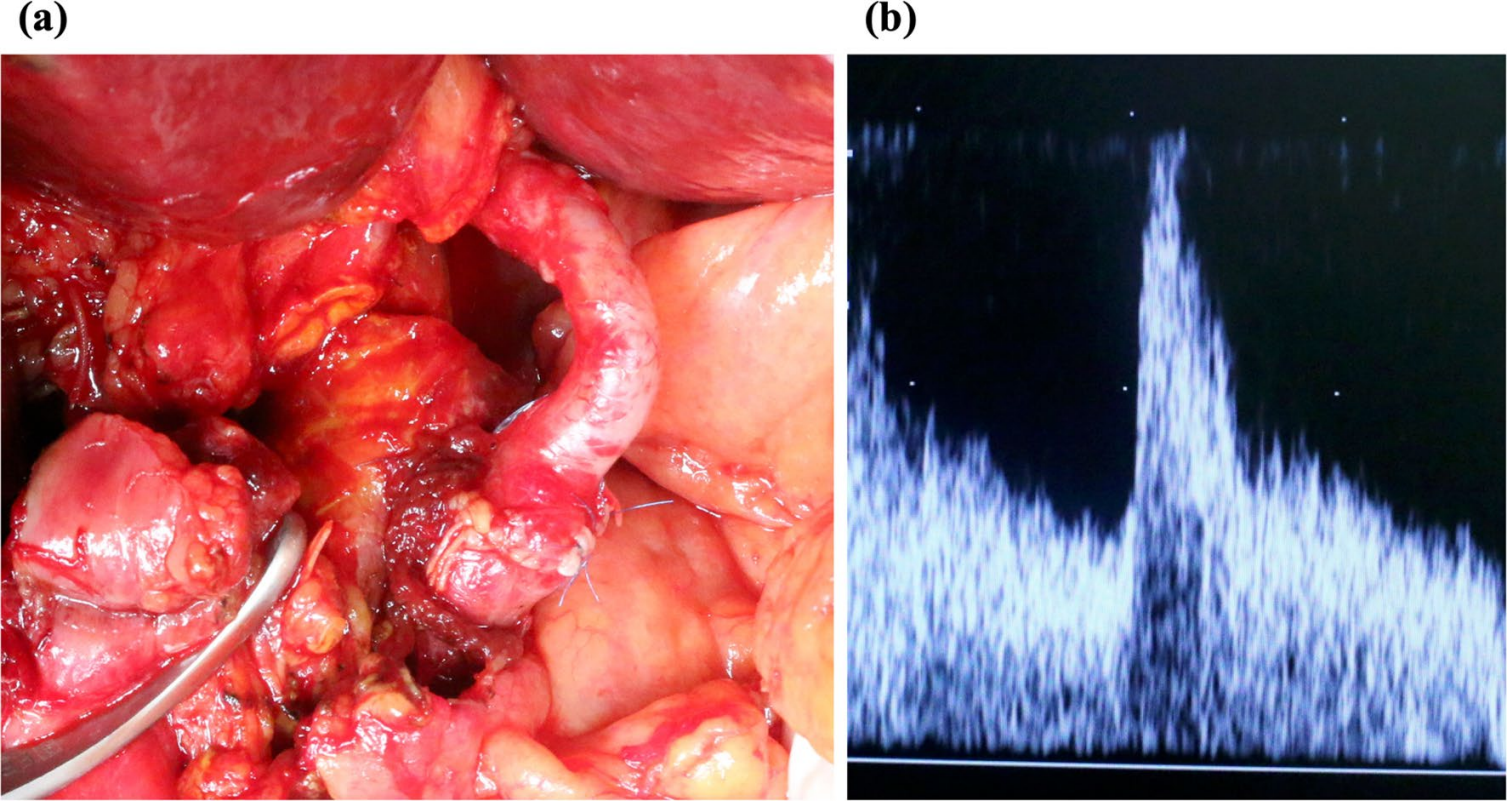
a Anastomosis of the recipient’s common hepatic artery with the graft’s common hepatic artery. b Evaluation of blood flow in anastomosed arteries by Doppler ultrasound
